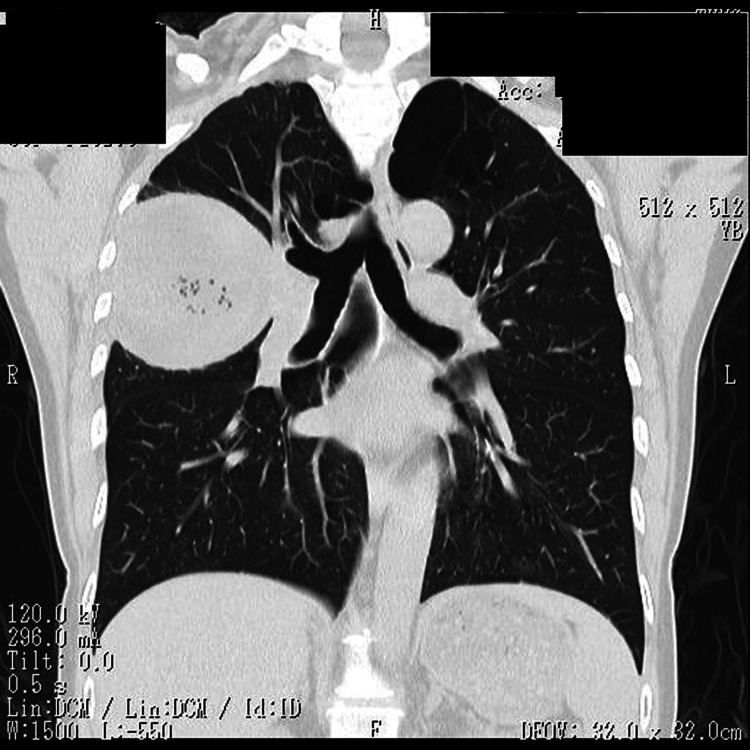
Chest CT at the time of clinical deterioration Development of an air-fluid level within the right upper lobe cyst, suggesting abscess formation.
